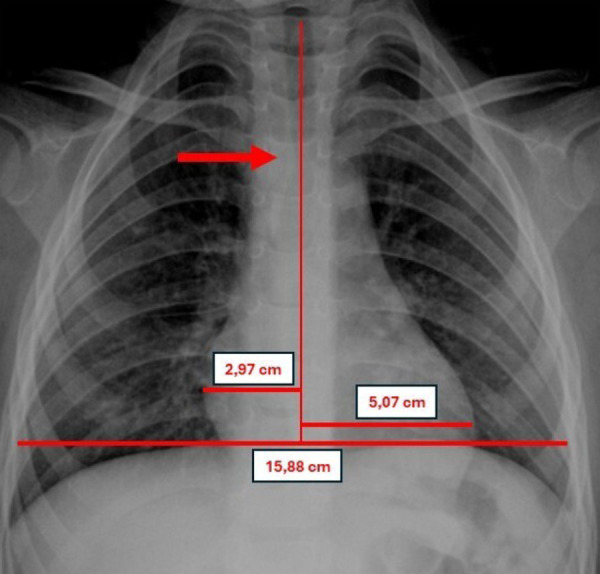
Anteroposterior chest radiograph showing preserved pulmonary vascularity, no evidence of cardiomegaly (cardiothoracic ratio: 0.50), and a notch in the mid-to-distal third of the trachea. CTR: cardiothoracic ratio.
